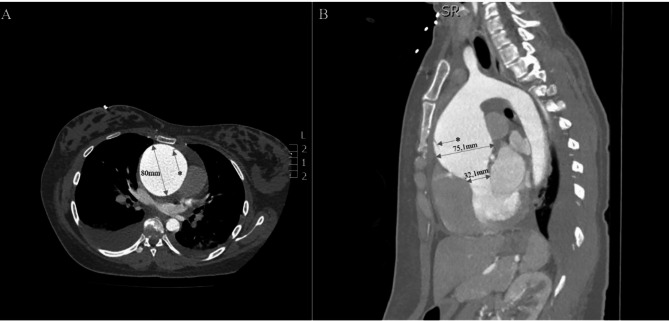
Preoperative CT angiography axial (A) and parasagittal (B) views depicting the intraluminal intimal flaps (marked with an asterisk) and the significant size of the post-dissection aneurysm of the ascending aorta
